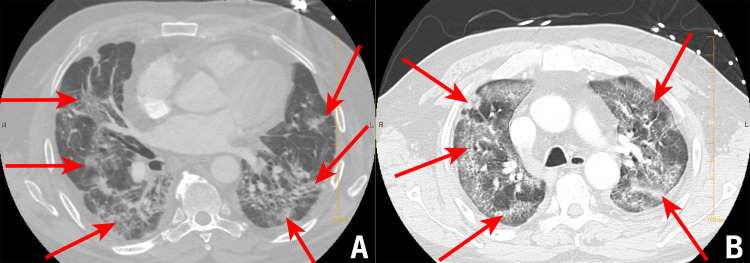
CT angiogram performed at the time of admission A and B: CT angiogram demonstrating fibrotic changes with reticulations, traction bronchiectasis, and areas of "crazy paving" ground-glass opacities consistent with sequelae of COVID-19 pneumonia CT: computed tomography; COVID-19: coronavirus disease 2019
